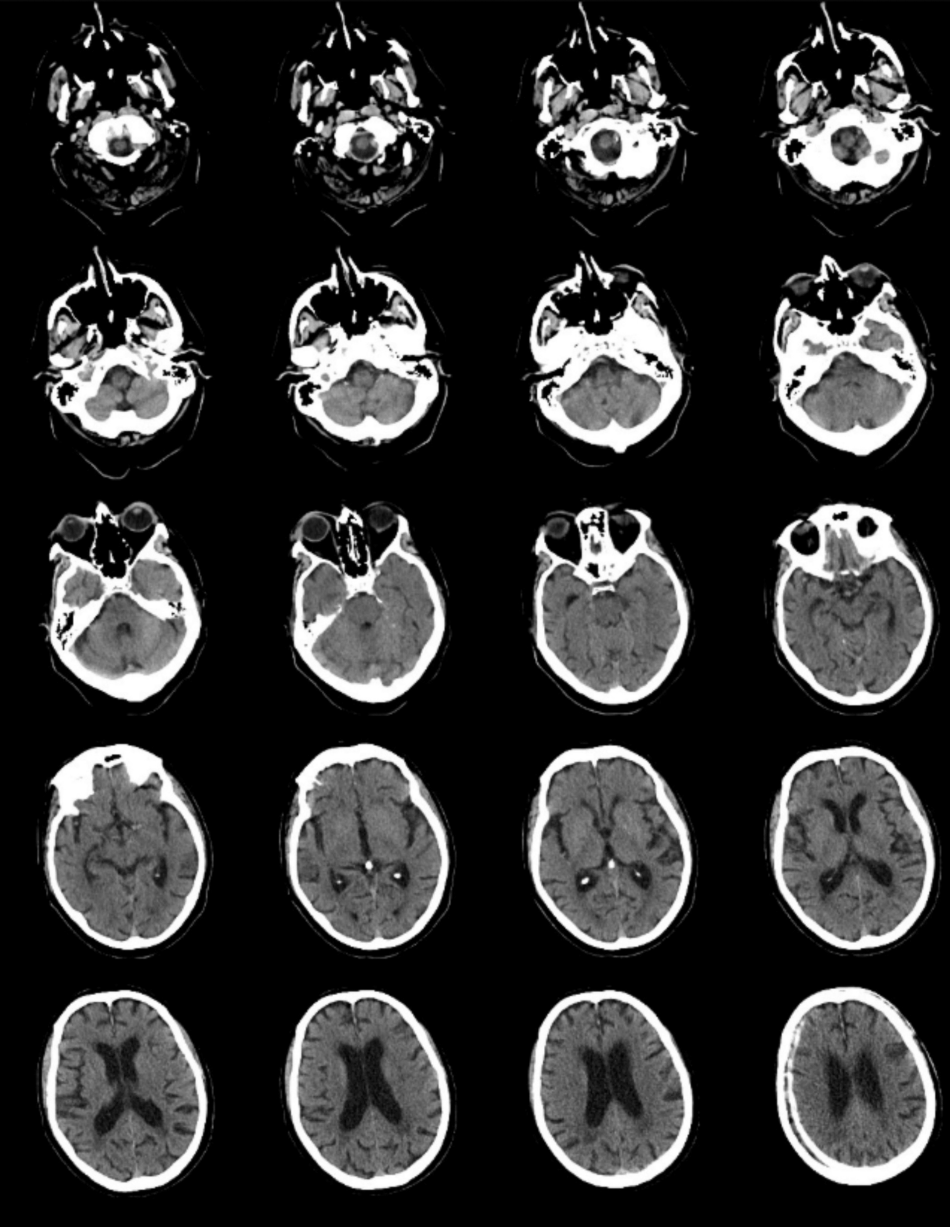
Axial sections of unenhanced computed tomography (CT) of the head showing no acute pathology.
